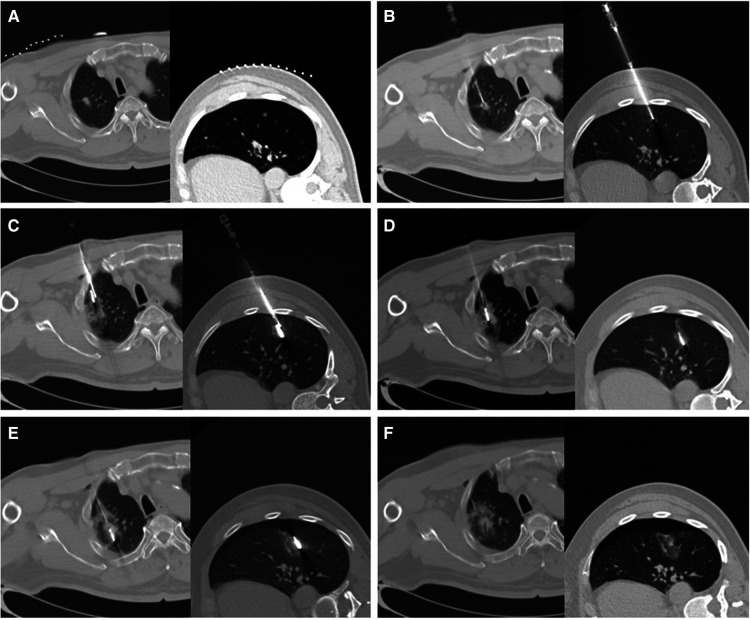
Case presentation. A 64-year-old female, presented with solid nodules in the right upper lobe and ground glass nodules in the left lower lobe. (A) Axial CT images of nodules in the upper lobe of the right lung and the lower lobe of the left lung. (B) Under the guidance of CT, the needle was inserted into the target site of the nodules, and the samples were cut and sampled. (C) Under the guidance of CT, the ablation needle was entered into the target site of the nodule, and the two needles were in the same field of vision. (D) After pre-ablation, the samples were cut again, and the CT images were reviewed. (E) After adjusting the ablation parameters, continue the ablation until the ablation range is satisfactory. (F) Immediately after the end of the ablation, CT review showed a little bleeding and pneumothorax.
